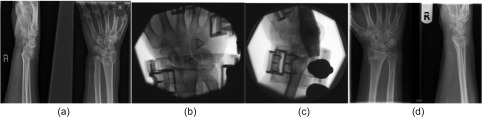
Radiographs and fluoroscopic images of a 65-year-old patient with unstable extra-articular DRF (a) treated with non-invasive dynamic external fixator (b and c). There was no collapse or shortening at the fracture at six-month post-op radiographs (d).
